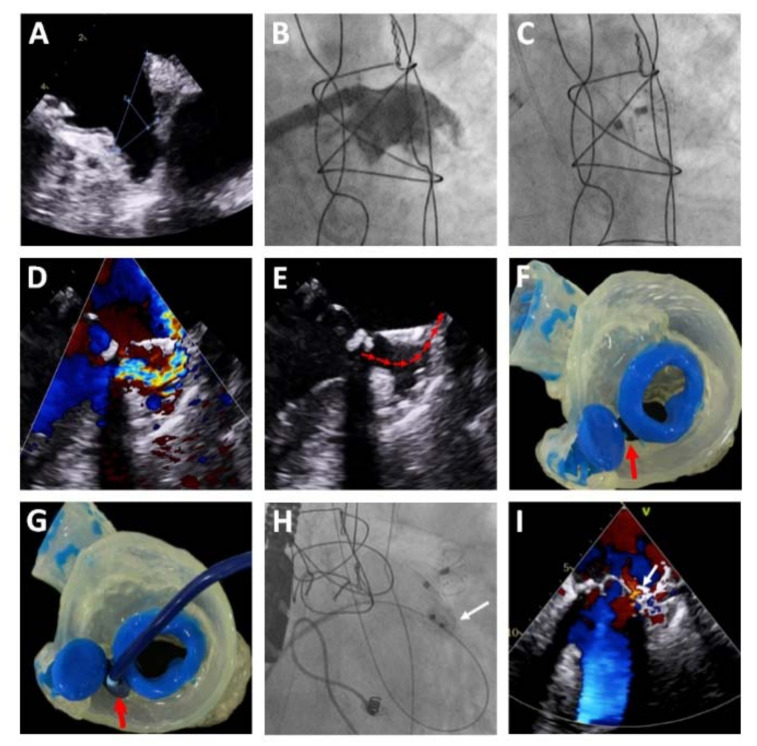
Case of left atrial appendage closure (LAAC) with the LAmbre™ device showing the resistance of the device to direct impact from a severe regurgitation jet from a mitral periprosthetic leak. Legend: (A–C) device implantation. (D,E) transesophageal echocardiography (TEE) showing a regurgitation jet from the leak toward the device, flowing between the cover disk and the umbrella (red arrow). (F,G) 3D model for planning of the percutaneous closure of the leak (red arrow). (H) Percutaneous closure of the leak with a 14 × 5 mm Amplatzer Vascular Plug III device (Abbott Vascular, USA) (white arrow). (I) TEE confirming the correct implantation of the device (white arrow) with no interference with the LAAC system or with the cusps of the mitral bioprosthesis, reducing the regurgitation to mild.
